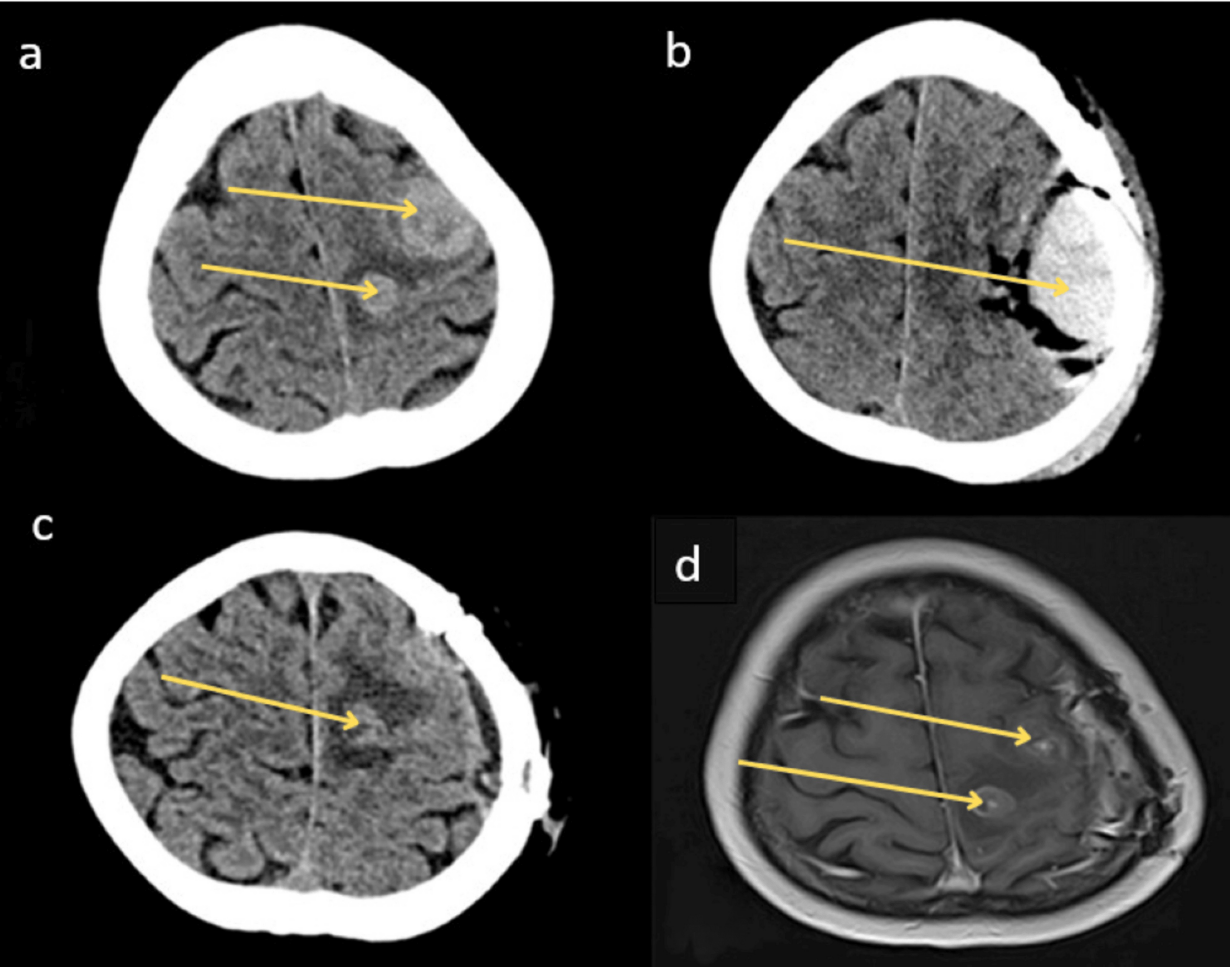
Timeline of Neuroimaging Findings in a Patient With Neurocysticercosis and Concomitant Metastatic Disease (a) Non-contrast head computed tomography (NCHCT) obtained during the patient’s initial emergency department visit shows two hyperattenuating parenchymal lesions within the left frontoparietal lobule (arrows), initially suspected to represent metastatic disease. (b) Post-operative NCHCT, one day after the initial craniotomy, shows resection of the intra-axial lateral lesion (arrow) with a 2.2 cm extra-axial hematoma beneath the skull flap. The medial lesion remains unchanged. (c) NCHCT, approximately two months post-op during evaluation for recurrent seizure, reveals persistent left frontal vasogenic edema (arrow) with a 1.1 cm nodular density, initially presumed to be a retained intracranial abscess. (d) T1-weighted magnetic resonance imaging (MRI), performed approximately nine months after the initial presentation, demonstrates a slight enlargement of the left medial frontal convexity (arrows) enhancing lesion with a mild increase in vasogenic edema. The left lateral lesion remains stable.
